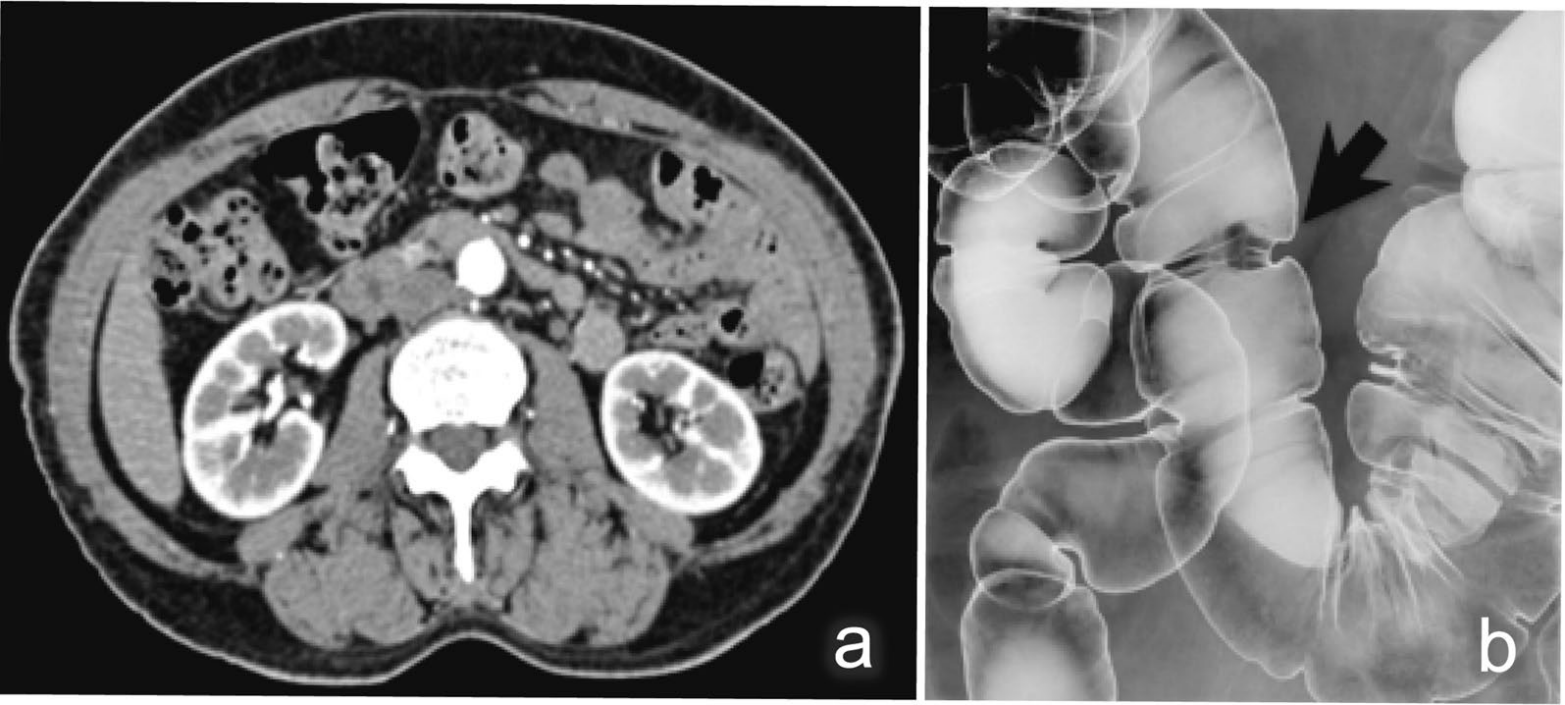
Individual imaging findings during the initial examination. a Computed tomography revealed no remarkable abnormality and no evidence of wall thickening in the transverse colon, lymph node metastasis, and distant metastasis. b Barium enema examination revealed an arc-shaped deformation in the transverse colon, confirming that the lesion was a type II tumor
